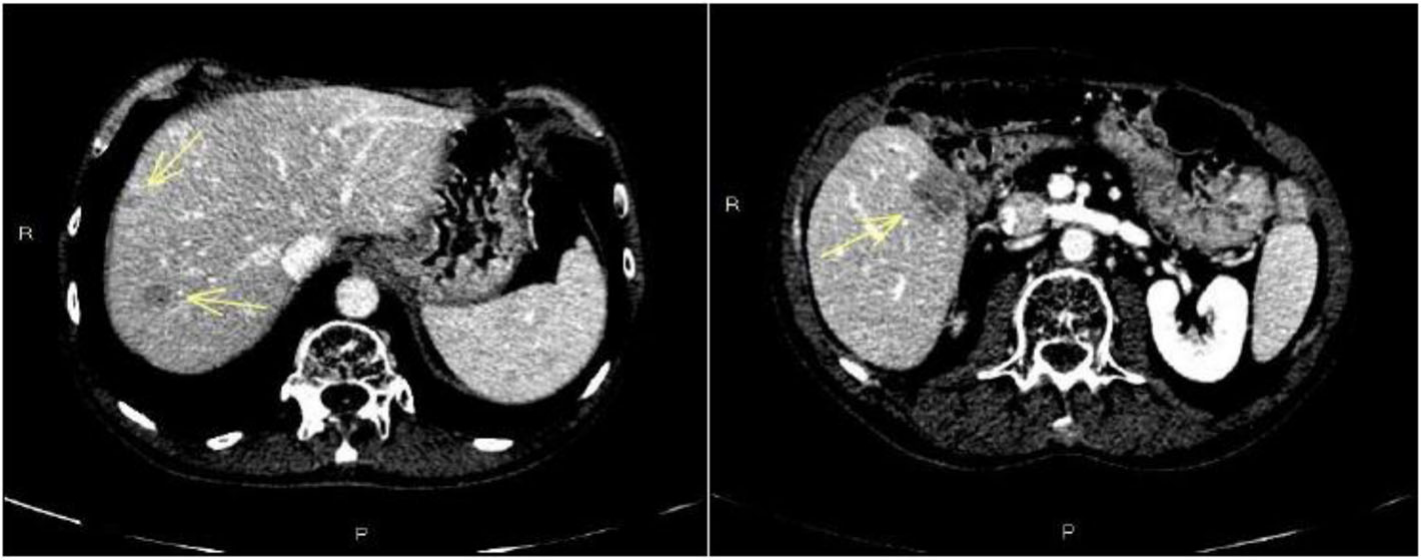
The abdomen CT scan highlighted the appearance of some secondary hepatic lesions (yellow arrows)
